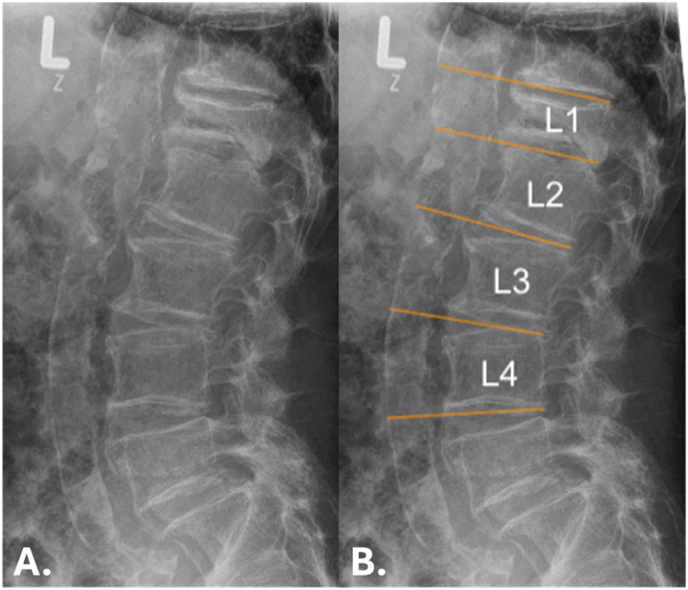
Assessment of abdominal aortic calcification (AAC) using the Kauppila scoring system. (A) Lateral lumbar radiograph. (B) Segmentation of the abdominal aorta adjacent to vertebral levels L1–L4. Calcification is evaluated separately along the anterior and posterior aortic walls at each vertebral level and graded from 0 to 3 based on the longitudinal extent of calcification. Segmental scores are summed to yield a total AAC score ranging from 0 to 24.
